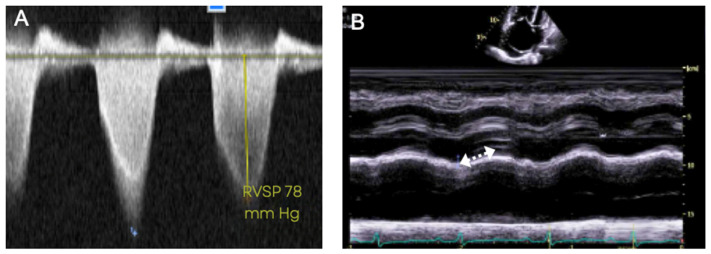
Doppler echocardiographic findings in pulmonary hypertension. The right ventricular systolic pressure (RVSP) can be estimated with the velocity of the tricuspid regurgitant jet by utilizing the modified Bernoulli equation: 4 X (velocity)^2^ + right atrial pressure. In the absence of pulmonic stenosis, the RVSP is equal to the pulmonary artery systolic pressure (A). Tricuspid annular plane systolic excursion (TAPSE) is obtained by placing the M-mode cursor in line with the tricuspid annulus. The degree of excursion is utilized to estimate right heart function (B). In this patient, the TAPSE is abnormal at 1.0 cm (normal > 1.8 cm).
